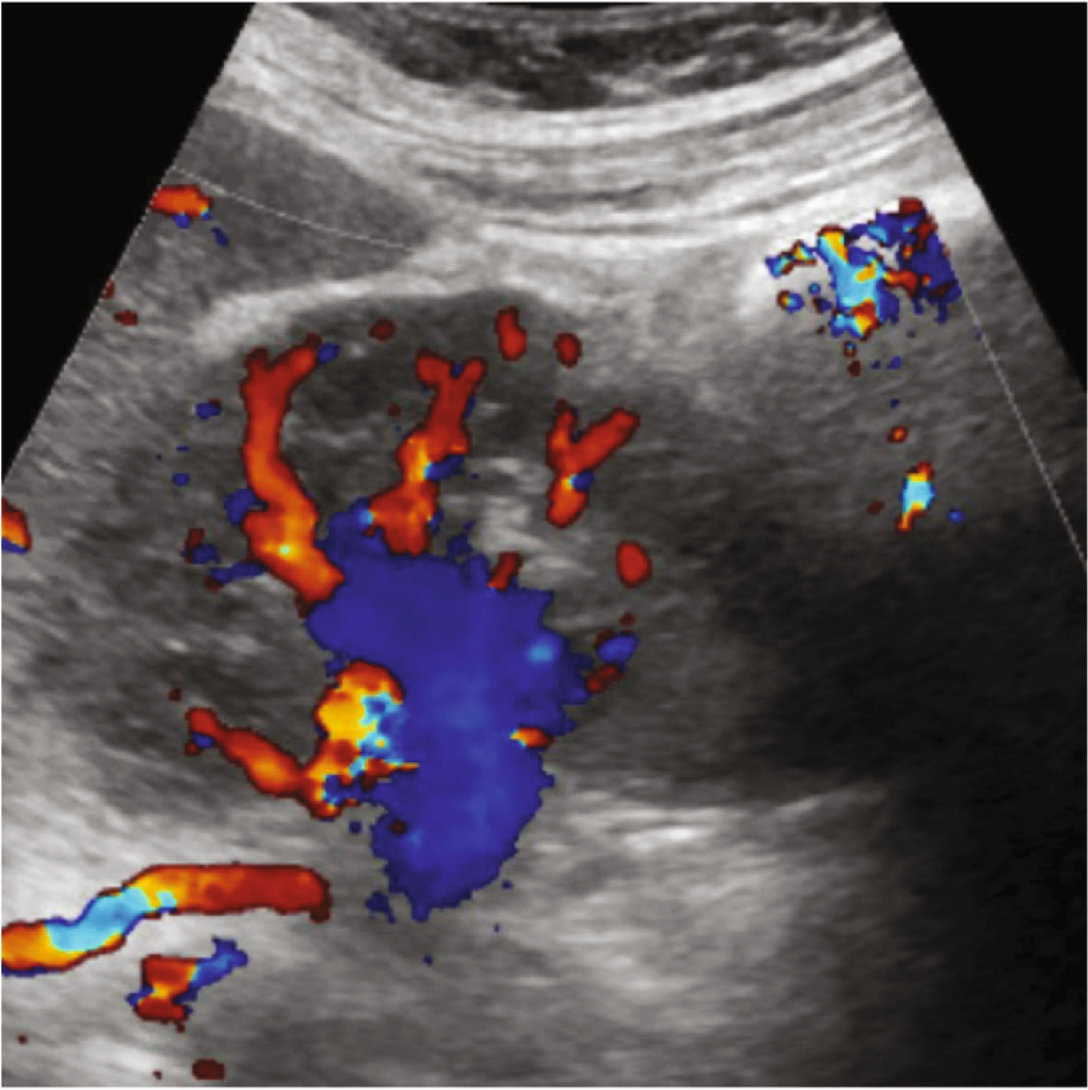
US color Doppler shows patent color flow in distal vessels.
